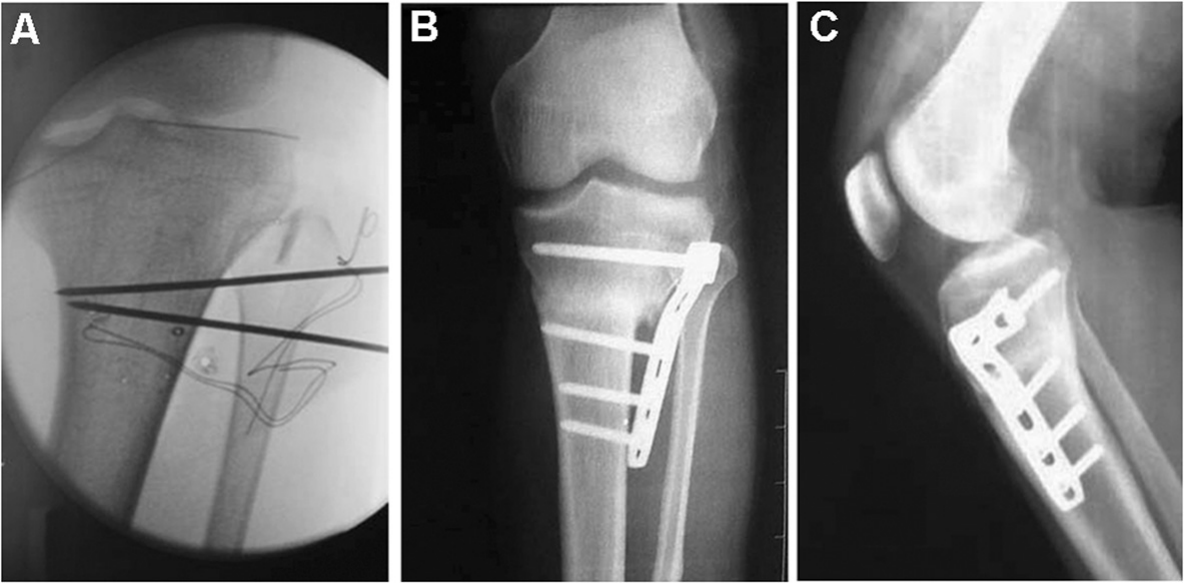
Modified procedure for CWHTO. The osteotomy site was at the distal third of the tibial tuberosity. The procedure was performed under fluoroscopy, and an LCP was inserted for internal fixation. (A) Two K-wires were intraoperatively drilled in as osteotomy guides. The proximal wire was inserted at the level of the distal third of the tibial tuberosity and parallel to the joint line. The distal wire was then inserted in order to indicate the desired angle of correction and exited through the medial cortex where the first wire exited. The angle was determined from preoperative radiographic assessments and confirmed by fluoroscopy. (B, C) AP and lateral radiographs, respectively, 9 months after operation. The proximal fixation screws were at the level of or immediately distal to Gerdy’s tubercle, far from the articular surface. A full contact between the plate and bone surface was not necessary.
